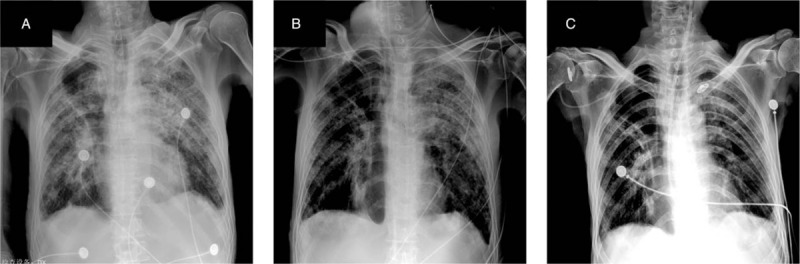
Chest X-ray examination of 64 years old man diagnosed with severe COVID-19 pneumonia. (A) On February 28, after tracheal intubation, chest X-ray showed interstitial inflammation of both lungs and a small amount of pleural effusion on both sides. (B) 7 days after tracheal intubation, chest X-ray examination showed multiple patchy increased density shadows in both lungs, which were more than before, and a small amount of pleural effusion on both sides was roughly the same as before. (C) Chest X-ray examination on March 28 before discharge showed multiple patches and strip like density shadows in double lungs, which were significantly improved than before. The right chest contains an air sac cavity.
